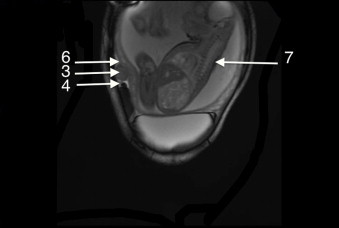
Abdominal Magnetic Resonance Imaging-T2 was performed and revealed uterine fibroid in Spigelian hernia (arrow). (3) Spigelian hernia. (4) Linea Semilunaris. (6) Uterus. (7) Fetus.
